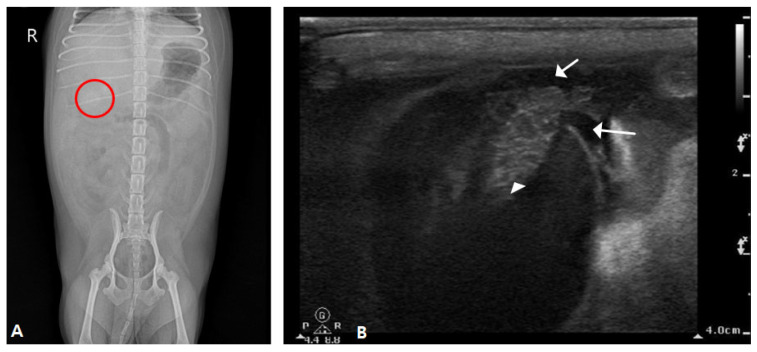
Radiography and sonography of the gallbladder. (A) An opaque material with rounded margins was identified in the right upper abdomen (round circle). (B) Hyperechoic round-shaped immobile sludge inside the gallbladder penetrated the abdominal gallbladder wall (arrowhead) and spanned the wall, and free fluid was identified around the gallbladder (arrow).
